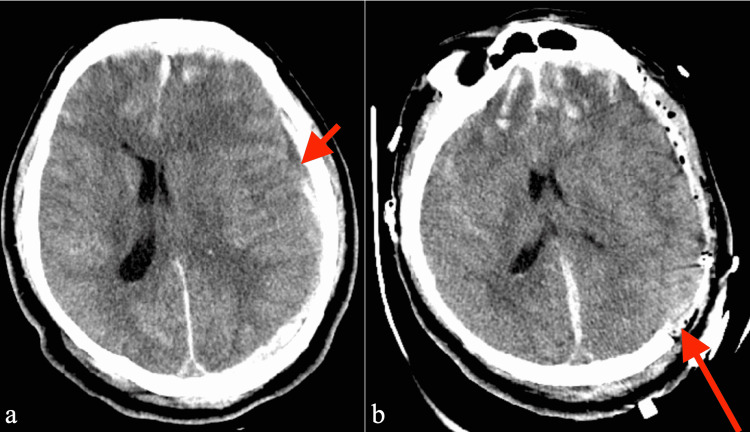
Preoperative (a) axial CT head showing acute/hyperacute left convexity subdural hematoma (short red arrow) causing left-to-right midline shift with compression of the left lateral ventricle. Bifrontal contusions were also demonstrated. Postoperative (b) axial CT head demonstrating large decompressive craniectomy (long red arrow) with the evacuation of subdural hematoma and improvement in midline shift.
